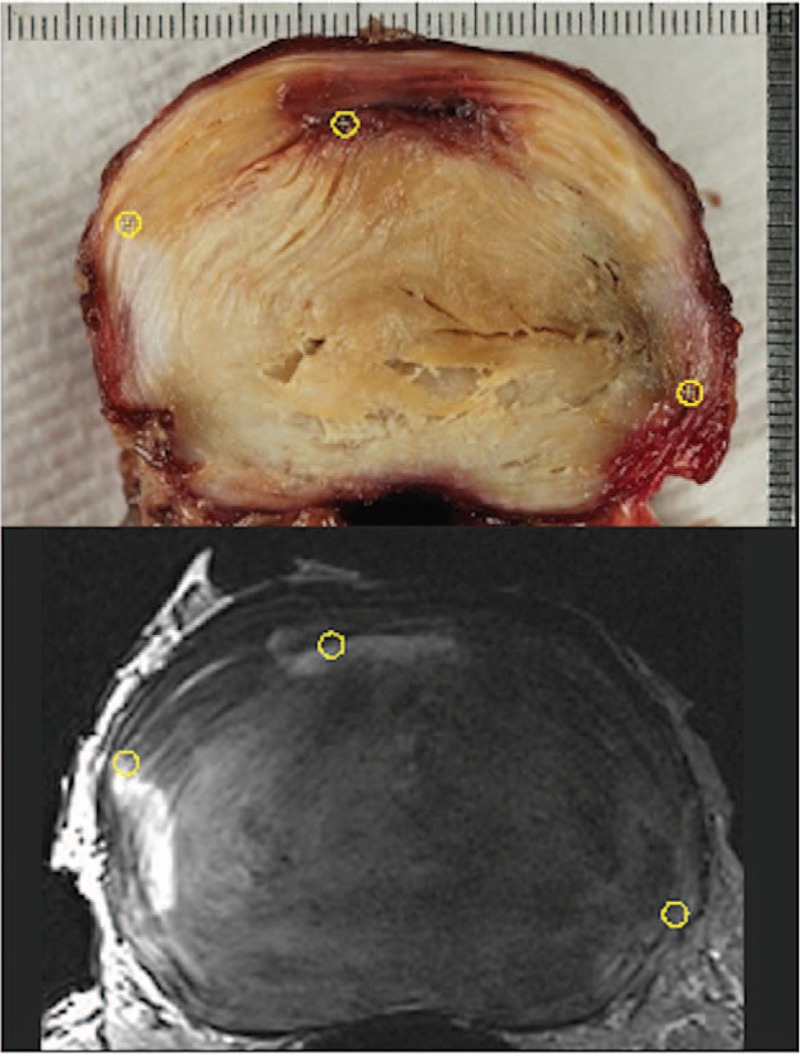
Example comparison of landmarks from an MR image (in this case T2-weighted-TSE) and corresponding cross-sectional photograph of a L2L3 IVD. On the MR image, the fluid build-up, vascularization, and the granulation tissue proliferation due to fissures are indicated well and clearly distinguishable from the surrounding tissue. IVD indicates intervertebral disc; TSE, Turbo-Spin-Echo.
